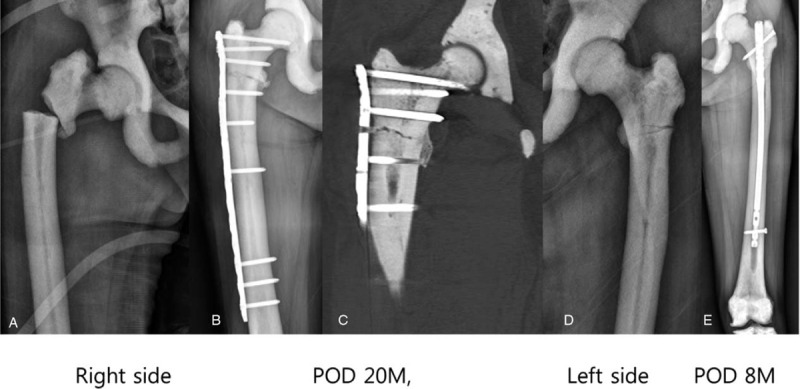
Bilateral subtrochanteric fractures in a 70-year-old woman with autosomal dominant type II osteopetrosis. A and D. A transverse subtrochanteric fracture at the lower level of the lesser trochanter in the right femur and incomplete fracture in the left femur are shown. B, C. Open reduction and plate fixation were performed on the right side. The peripheral callus is visible on plain radiography and computed tomography at 20 months postoperatively, but the fracture line remained because of delayed bone remodeling. E. The incomplete fracture line disappeared 8 months after prophylactic nailing on the left side.
